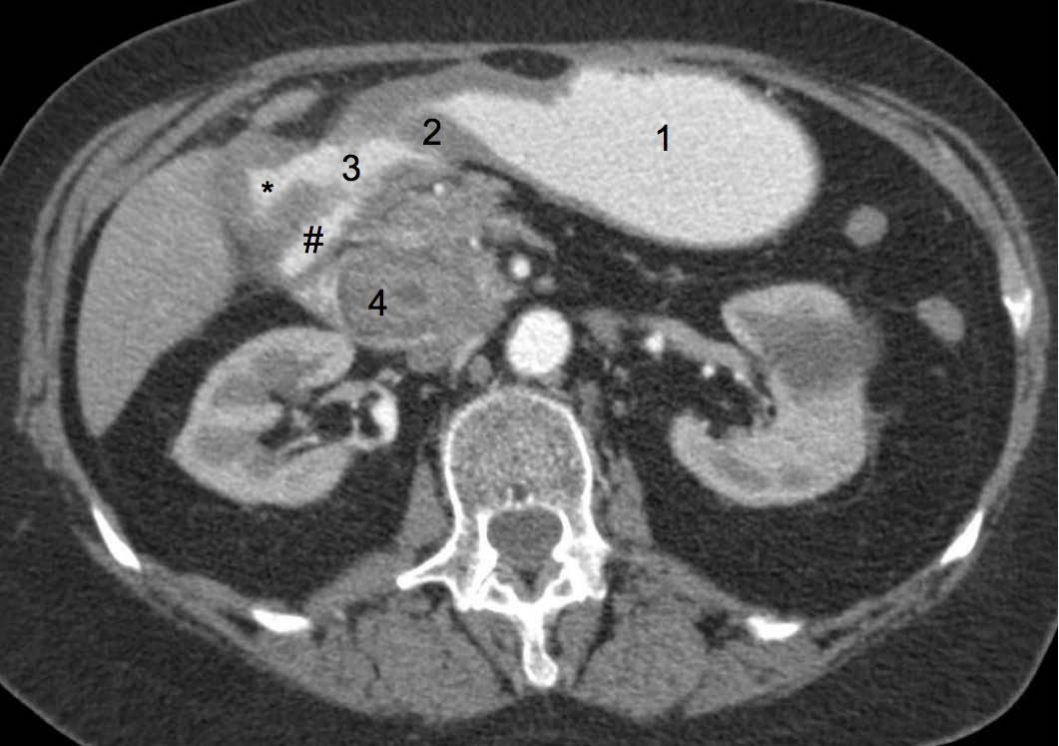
Abdominal computed tomography (CT) scan with intravenous and oral contrast enhancement. Labels on the figure are as follows. 1: Contrast material in the lumen of the stomach. 2: Pyloric sphincter. 3: Contrast material in the lumen of the duodenal bulb. 4: Gallstone impacted in the lumen of the third part of the duodenum. *: Contrast material in the lumen of the gallbladder (notice thickening of the wall of the gallbladder and communication with the duodenal bulb). #: Contrast material in the lumen of the descending duodenum.
